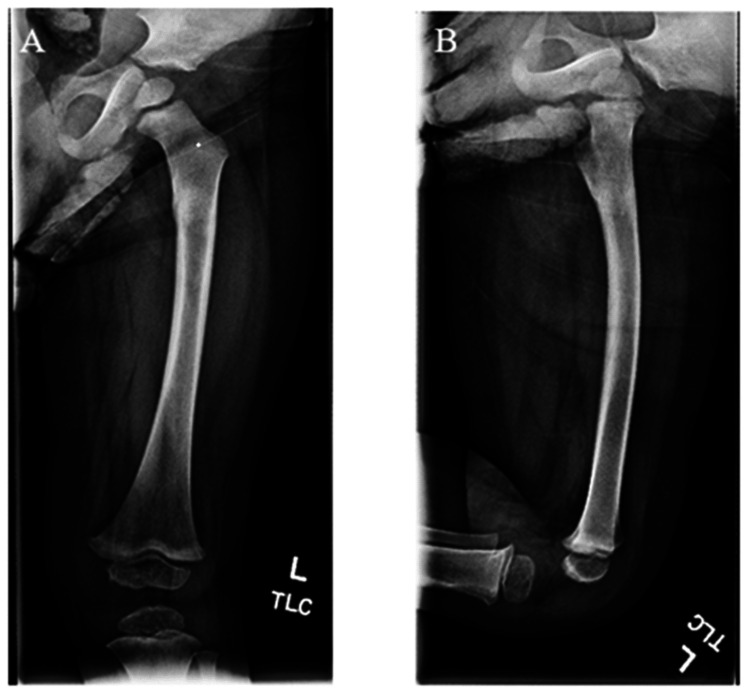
Final imaging for case 2, including (A) AP and (B) lateral radiographs of the left lower extremity demonstrating the healing of the subtrochanteric proximal femur fracture
